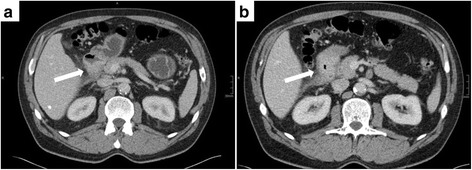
Computed tomography (CT) scans (a/b) of a duodenal pancreatic heterotopia (arrows) with a duodenal stenosis
